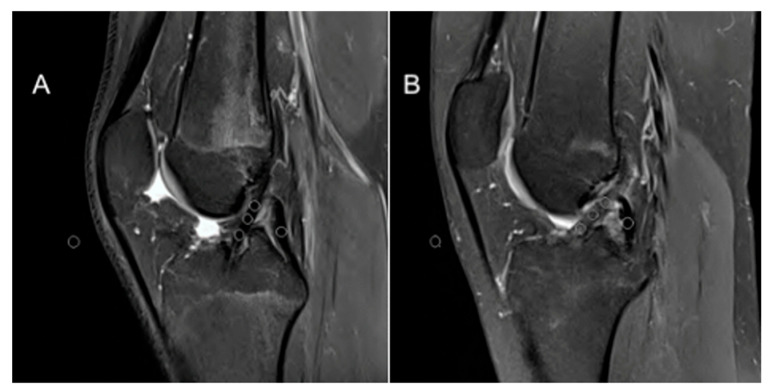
Measurement of SNQ on a sagittal T2 FS MRI. (A)—SNQ = 1.2 and Howell grade = I. (B)—SNQ = 9.4 and Howell grade = III. SNQ, signal-to-noise quotient; FS, fat saturation; MRI, magnetic resonance imaging. Circles : Placement of regions of interest (ROIs) : 3 ROIs on the intra-articular segment of the anterior cruciate ligament (1 proximal, 1 middle, 1 distal), 1 ROI 2 cm in front of the patellar tendon and 1 ROI on the posterior cruciate ligament.
